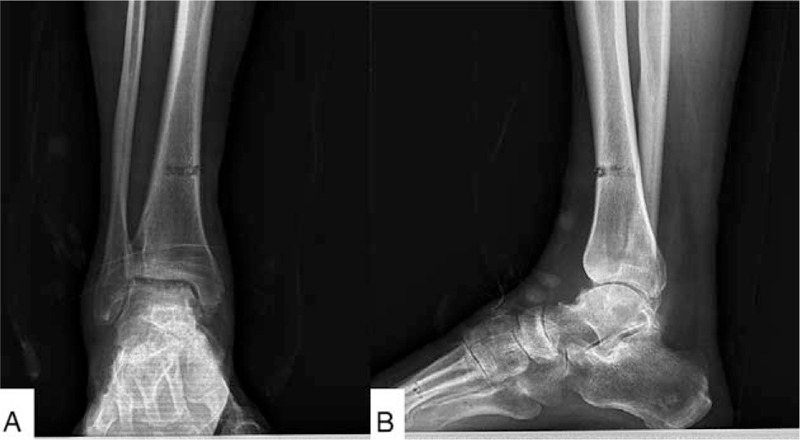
The weight-bearing radiograph showed that the joint space remained clear 3 mo after the external fixator was removed.
